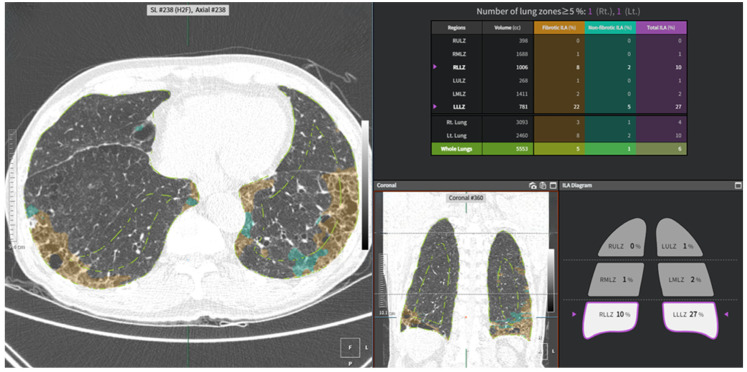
A user interface of an automated quantitative tool for interstitial lung abnormality (ILA). The system divides both lungs into upper, middle, and lower zones based on the levels of the inferior aortic arch and right inferior pulmonary vein (white dash line). Fibrotic ILA (orange), non-fibrotic ILA (mint), and total ILA (purple) extents are quantified in each zone and visualized on the CT images and in the summary table. Representative non-contrast chest CT images of a 75-year-old male smoker with subpleural fibrotic ILA (under the green line) demonstrate subpleural traction bronchiectasis with reticular opacities (orange) and ground-glass opacities (mint) in both lower lung zones.
